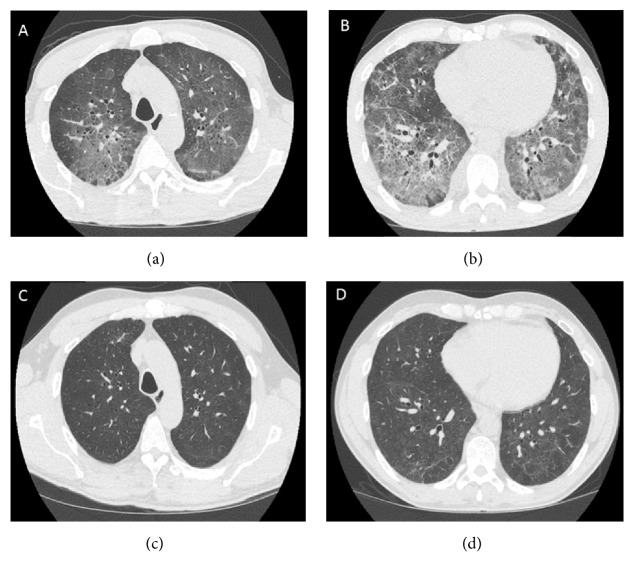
(a) and (b) At the time of presentation, axial computed tomography of the chest images show upper lobe predominant ground glass attenuation, mild interlobular septal thickening and small cysts. (c) and (d) Eight weeks following treatment, axial computed tomography of the chest images shows near complete resolution of ground-glass attenuation, interlobular septal thickening and cysts.
